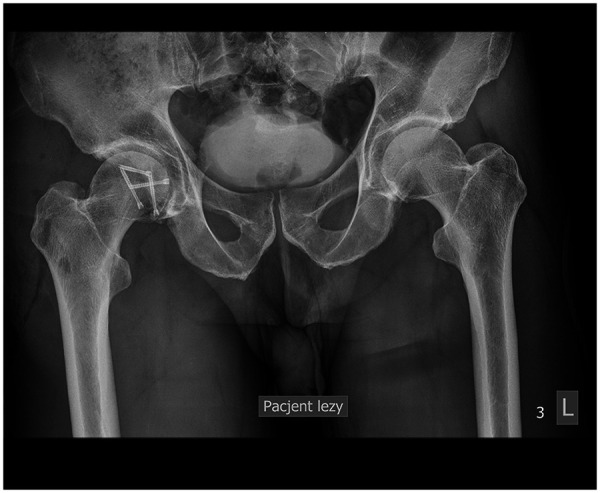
Anteroposterior radiographic image of hip joints on the first day after operation, demonstrating successful proper hip reduction with ORIF.
